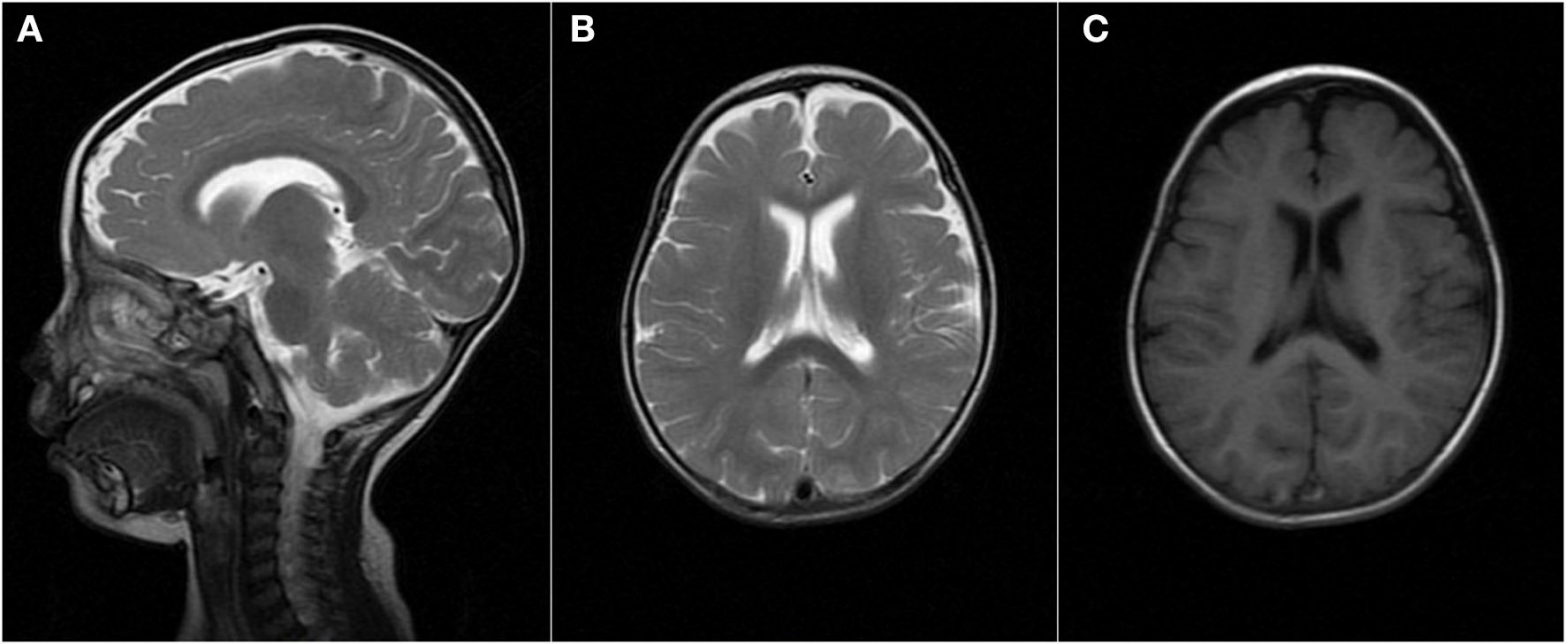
Brain MRI finding at the age of 11 months. MRI [(A,B) T2, (C) T1] demonstrated the widening of the extracerebral space at the bilateral temporal poles.
